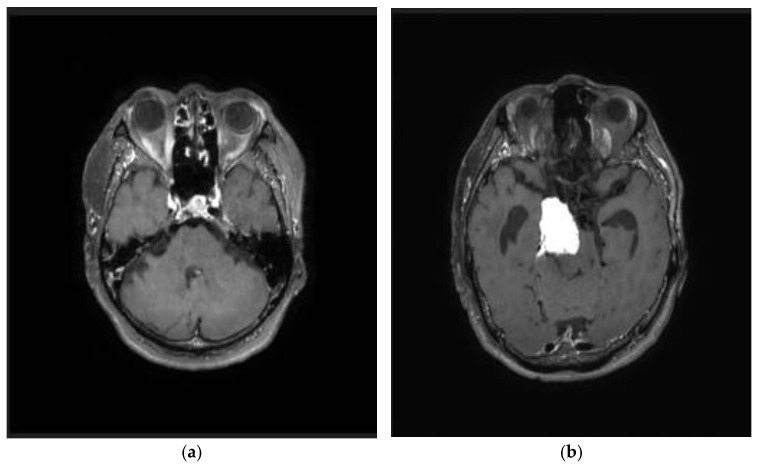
Normal (a) and abnormal (b) MRI data.
